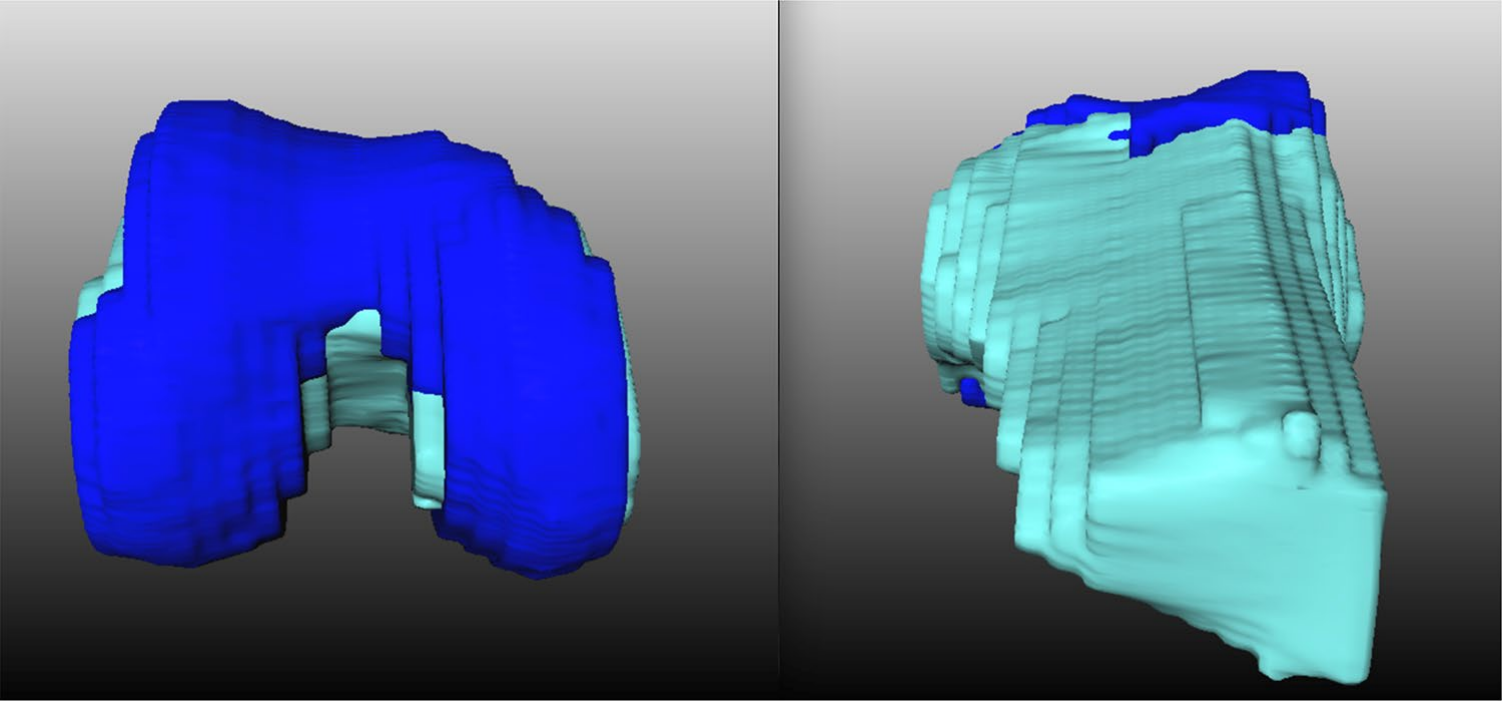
Software-generated MRI-based 3D model of the knee. Blue structure: representation of the femoral cartilage in a 3D model; Green structure: representation of the femoral bone in a 3D model; *Left* camera orientation: foot, *Right* camera orientation: head
